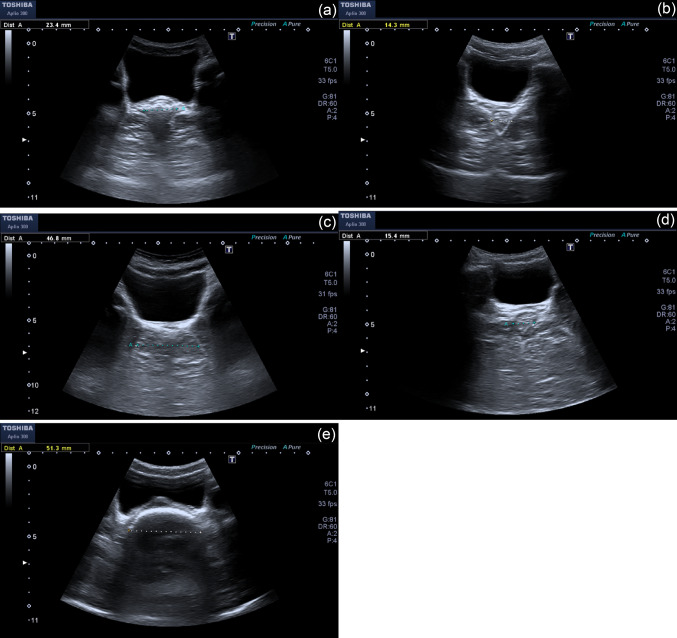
Visualizes the ultrasound measurements of the transabdominal transrectal diameter in a a healthy control without symptoms b a child with ARM and no symptoms c a child with ARM and symptoms, and d following successful bowel management, e a child with FC and ROM-IV criteria fulfilled
